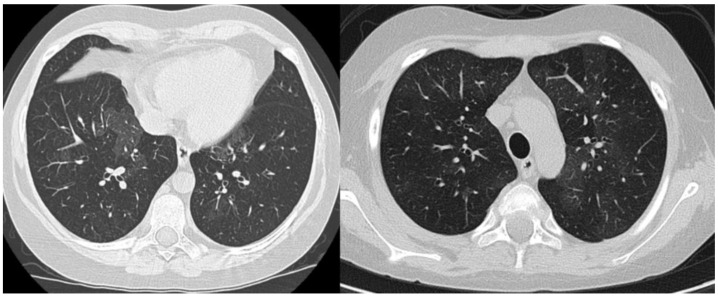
Pulmonary HRCT—transverse sections showing diffuse bilateral ground-glass opacities.
